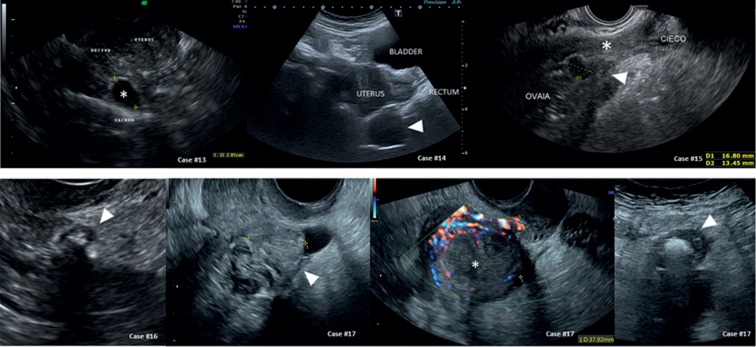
Images of multifocal bowel endometriosis (sonographic details of each case are reported in Table 3).
